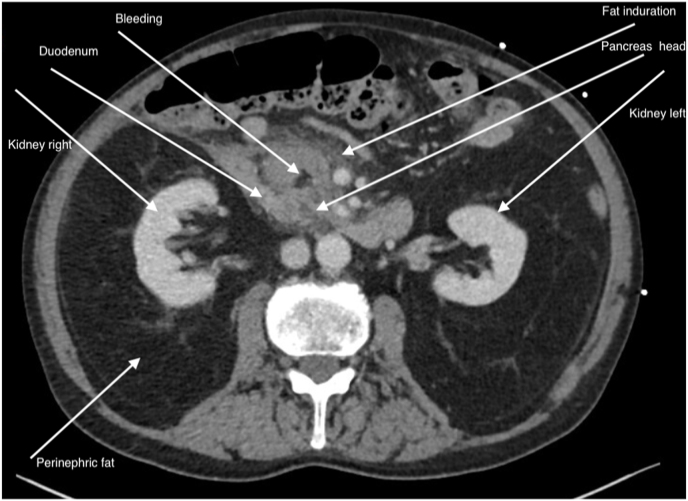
Transversal CT image: a bit fluid around the head of the pancreas.
